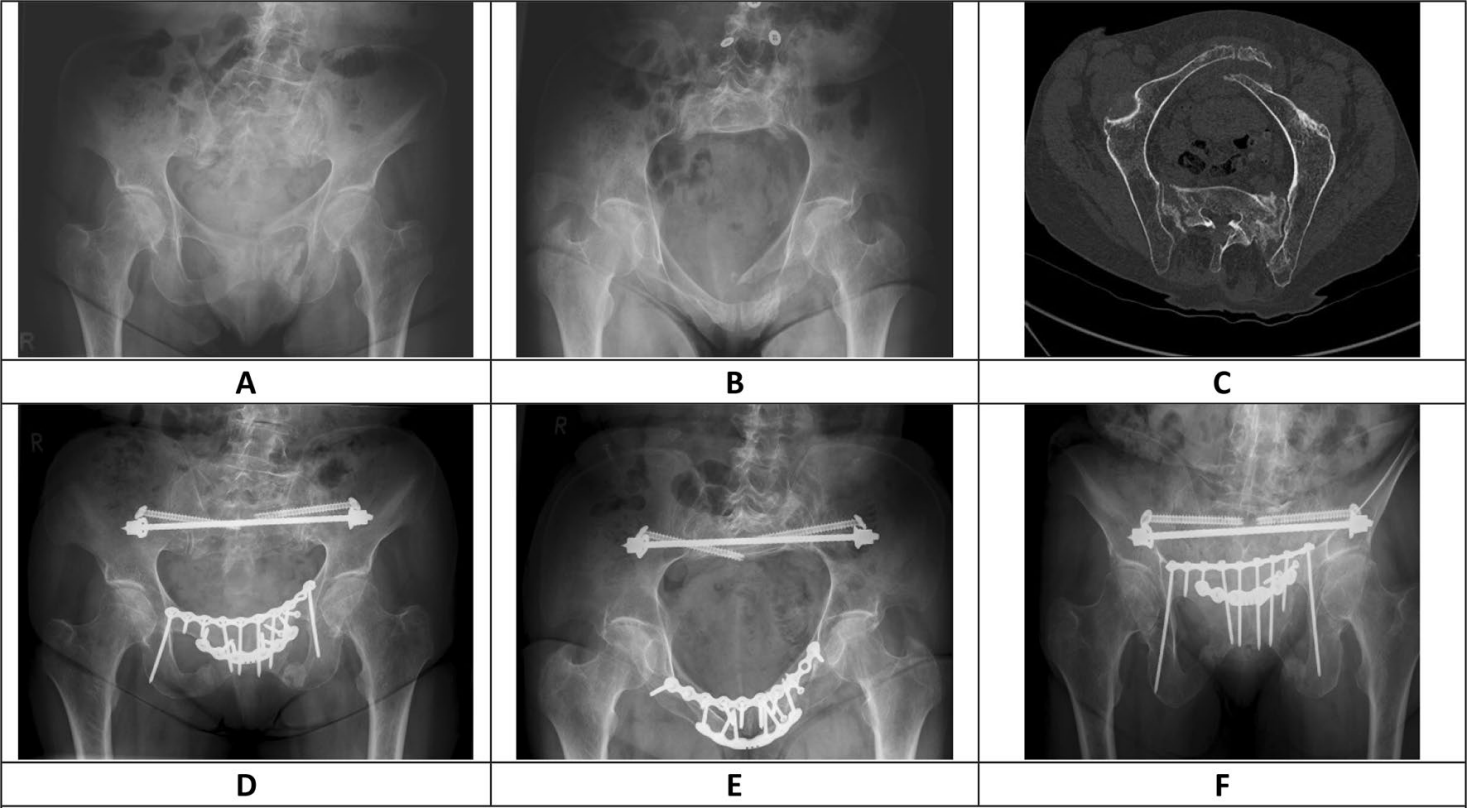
Shows an 83 year old female patient with a FFP 4b and a largely displaced fracture in the Nakatani II region of the anterior pelvic ring. (a Conventional ap radiograph, b conventional inlet radiograph, c CT reconstruction in the pelvic brim view). The posterior pelvic ring was treated with a sacral bar and bilateral SI screws. The anterior fractures was treated with a DPO (superior ten-hole non-angular stable plate with bilateral infra-acetabular screws, two lag screws, six-hole angular stable anterior plate). This patient did not have any screw loosening within the follow-up period of 12 months. (d Conventional ap radiograph, e conventional inlet radiograph, f conventional outlet radiograph)
